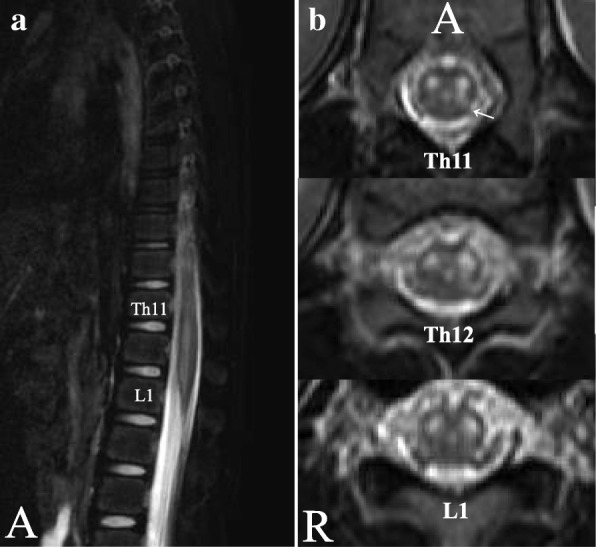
a Sagittal T2-weighted magnetic resonance image showing a hyperintense lesion and spinal cord edema continuously from the T11 to L1 spinal levels; b axial T2-weighted images at T11, T12 and L1 show hyperintense lesions in both anterior horns, worse on the left, with left posterior horn involvement (arrow). Key: R, right; A, anterior
